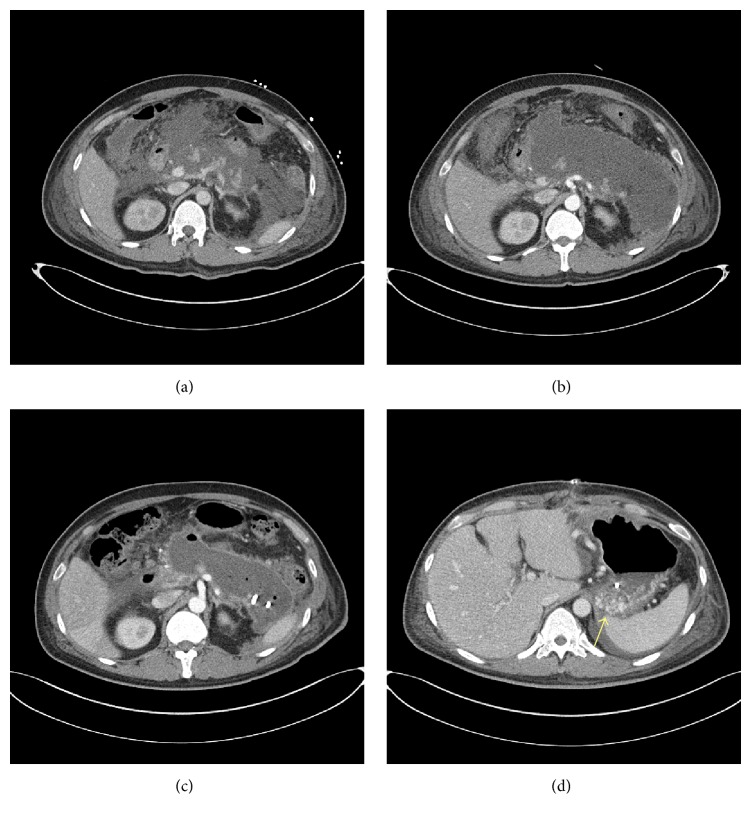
(a) Abdominal CT scan showed severe necrotizing pancreatitis. (b) Abdominal CT scan following 2 weeks of medical treatment. A huge pseudocyst was seen. A percutaneous drainage was performed. (c) Follow-up CT at 4 weeks after external drainage. The function of drain was poor and a huge pseudocyst was still seen. A necrosectomy and surgical drainage was performed. (d) CT scan at 1 week following the operation. Engorged submucosal veins were observed at gastric fundus.
